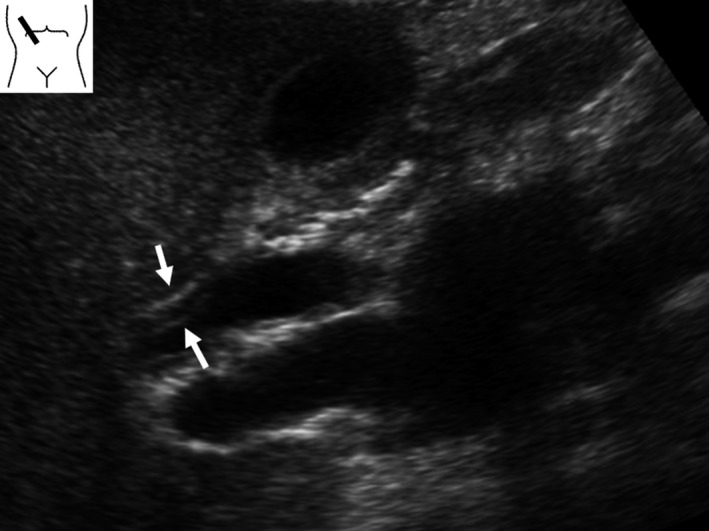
Ultrasonographic image of IgG4‐SC. Ultrasonography detects marked wall thickening in the extrahepatic bile duct (arrows)
